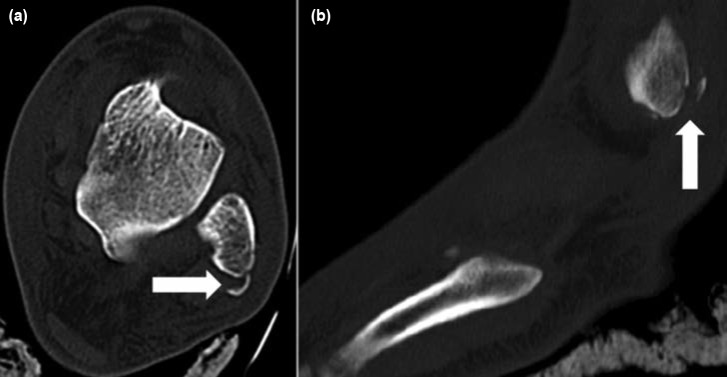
(a) Axial and (b) Sagittal CT scan show avulsion of superior peroneal retinaculum from the fibula.
